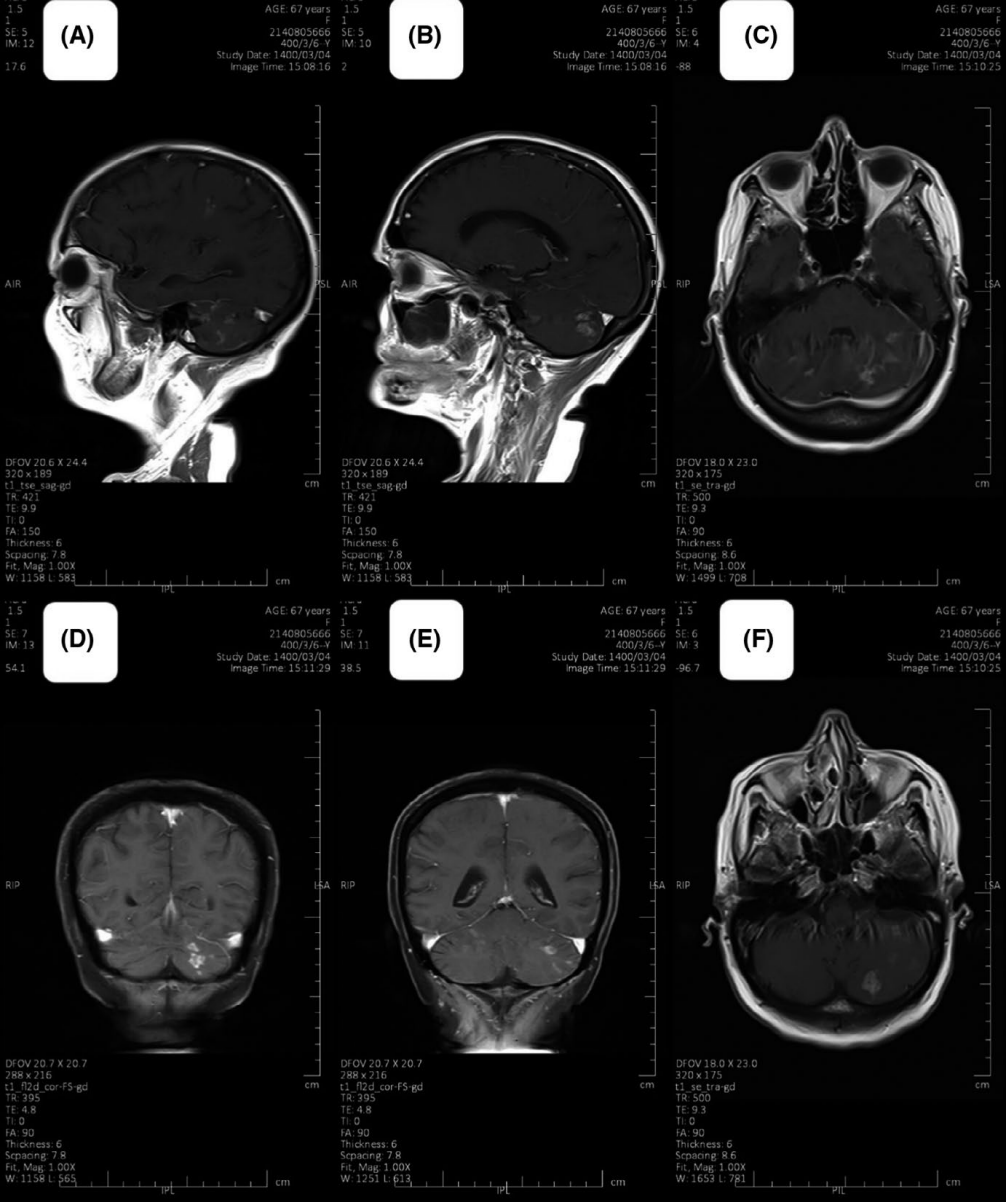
Brain MRI obtained in sagittal plane (A and B), axial plane (C and F), and coronal plane (D and E). On post‐contrast MR images, there are multiple highly enhancive foci at the cortical surface of both cerebellar hemispheres and posterior aspects of both parietal and occipital lobes, which all are suggestive of leptomeningeal metastases
